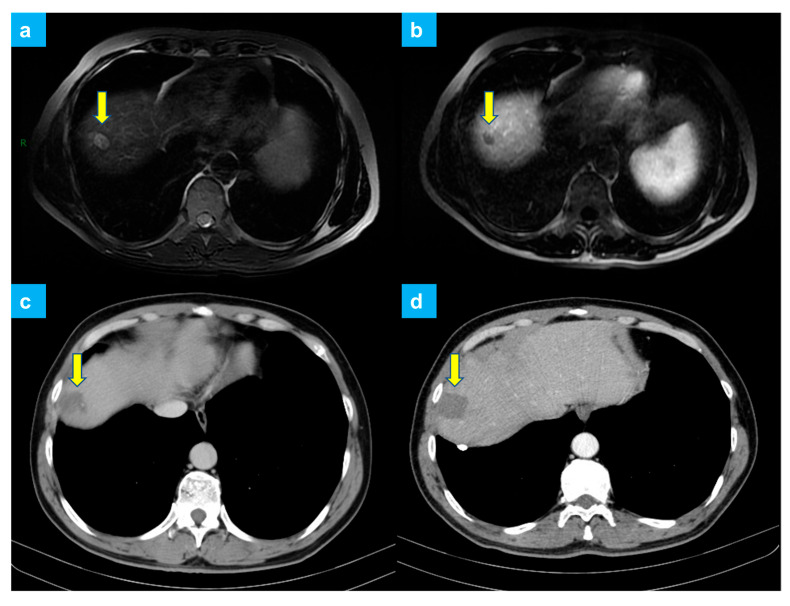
A case of TA for initially resectable CRLM. (a,b) Before TA, the enhanced MRI showed a slight ring enhancement (yellow arrow). (c,d) Forty days after TA, the enhanced CT showed that the ablation lesion in the upper part of the liver did not exhibit any significant enhancement (yellow arrow).
